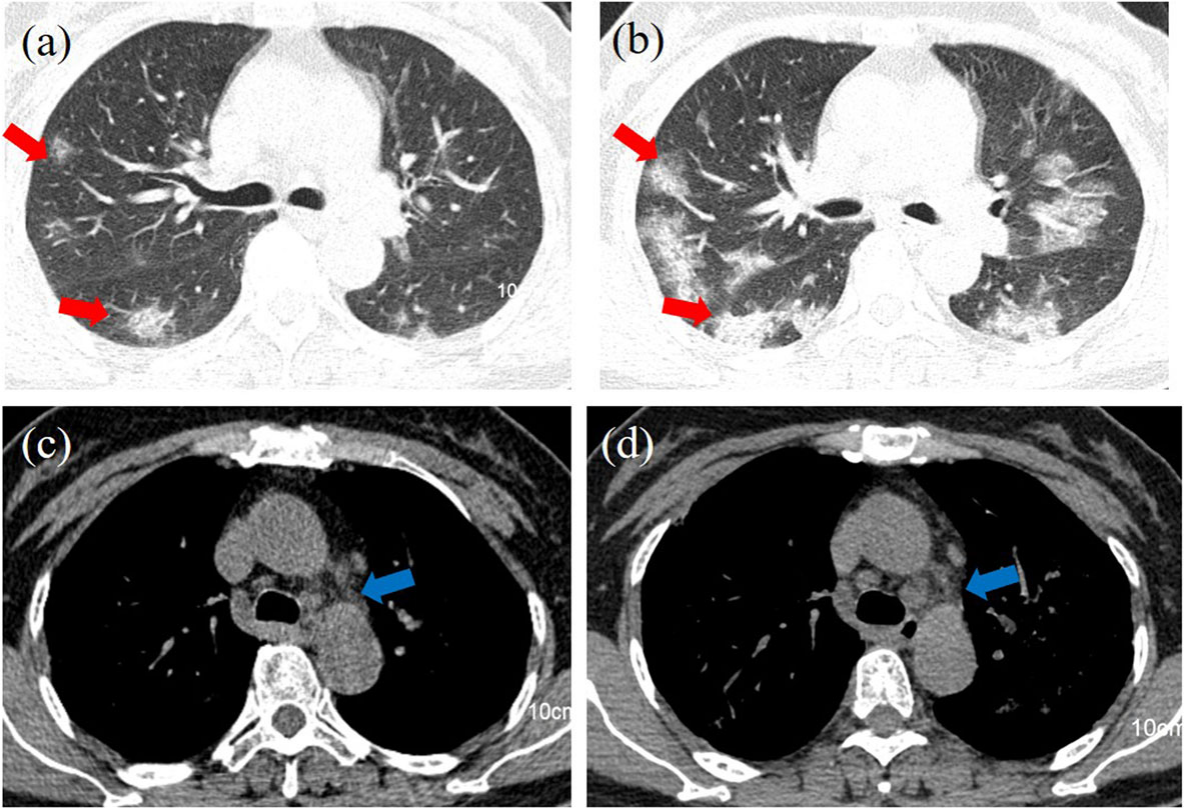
A female patient infected with SARS-CoV-2. The first CT scans (a) and (c) were performed four days after onset of symptoms. The second CT scans (b) and (d) were performed ten days after the treatment. Chest CT images showed the enlargement of ground glass opacity (red arrows) and intrathoracic lymph node (blue arrows) after 10-day treatment
